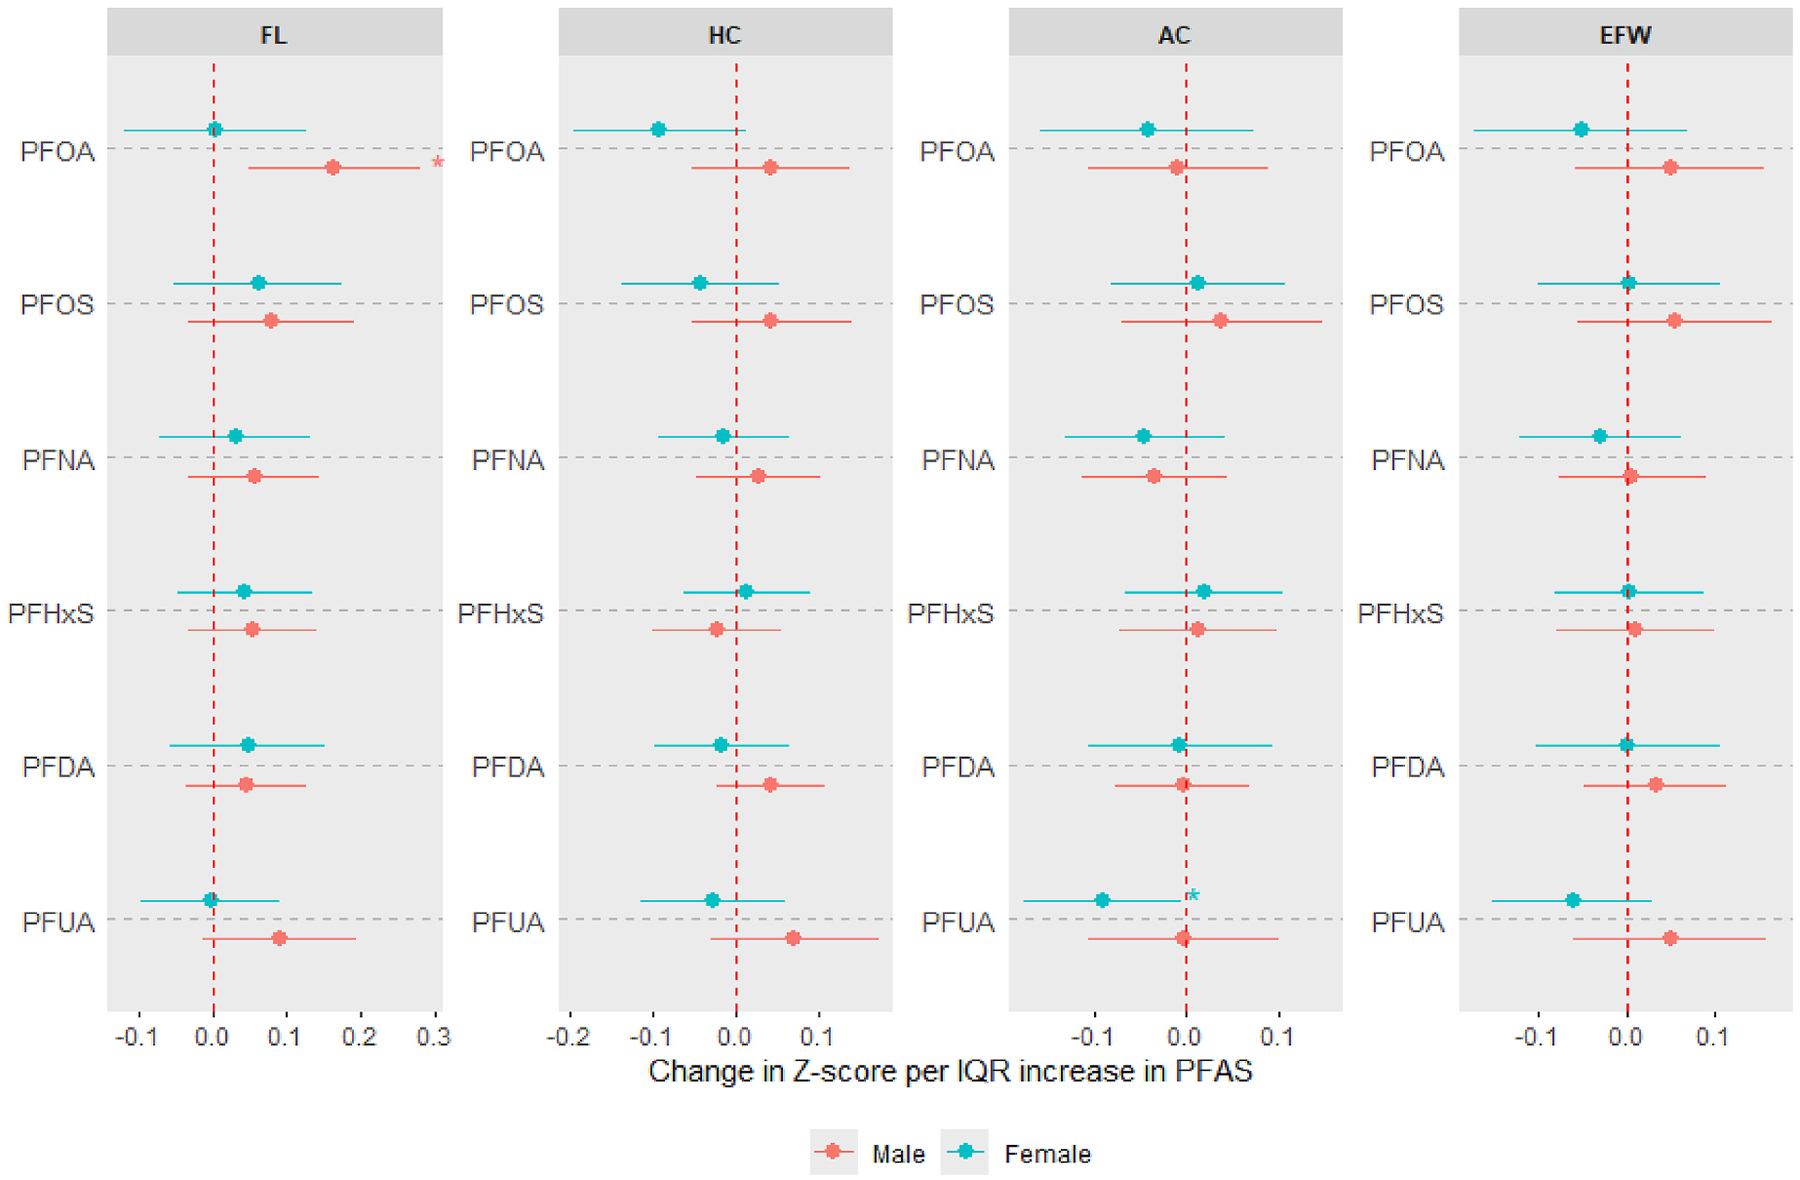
Associations between prenatal PFAS exposure and standardized ultrasound parameters of fetal growth [femur length (FL), head circumference (HC), abdominal circumference (AC) and estimated fetal weight (EFW)], stratified by fetal sex. Estimates derived from generalized estimating equations with exchangeable correlation. Effect estimates (95% confidence intervals) show the change in Z-score (or standard deviation) of each measure per interquartile range increase in log-transformed PFAS concentrations. Separate models were run for each sex. All models were adjusted for maternal age, prepregnancy BMI, race, education, insurance status, parity, enrollment year, and number of ultrasound scans. Asterisks indicate estimates with 95% confidence intervals excluding the null.
